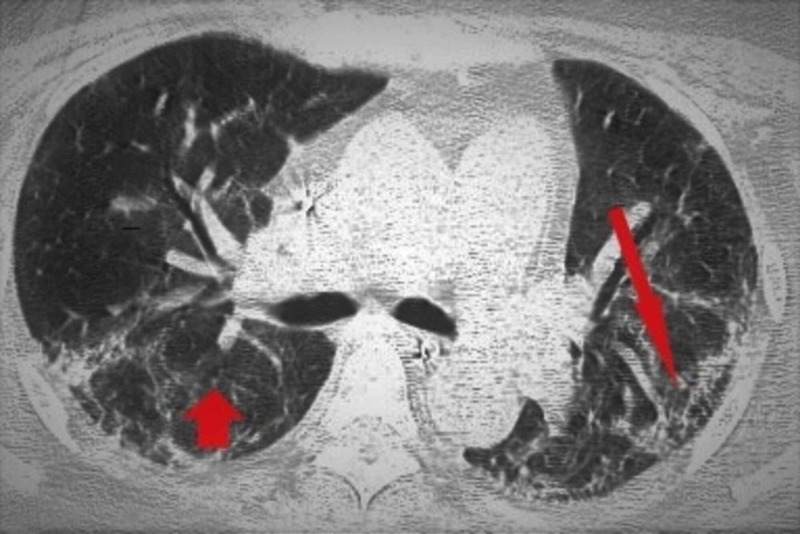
Thorax CT scan: bilateral interstitial pneumonia (arrows)
